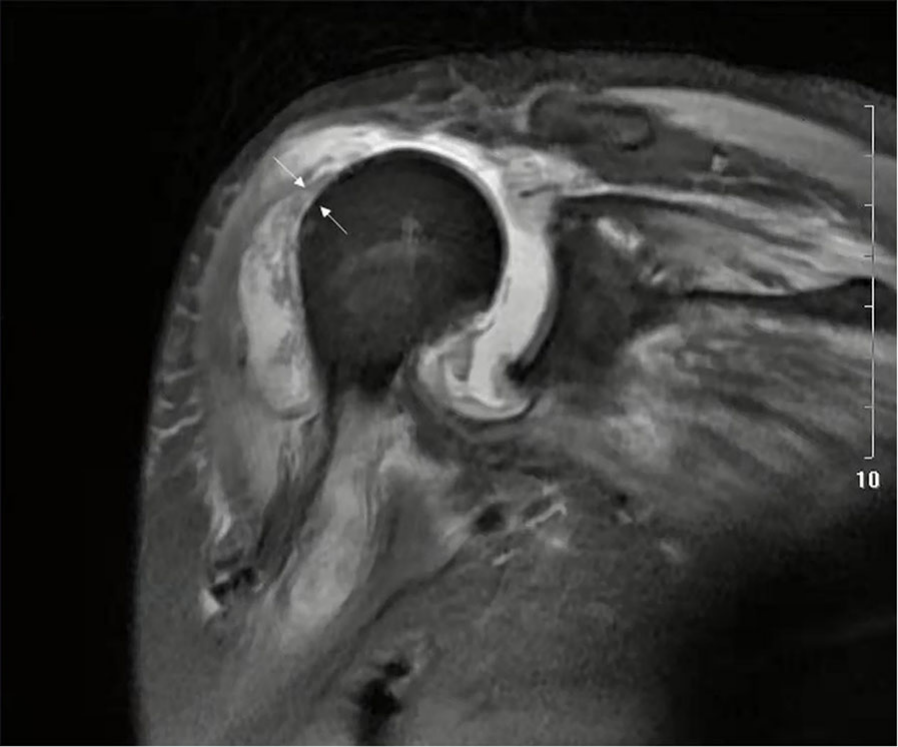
MRI of the right shoulder showing supraspinatus tendon tear (arrow) and massive abscess
